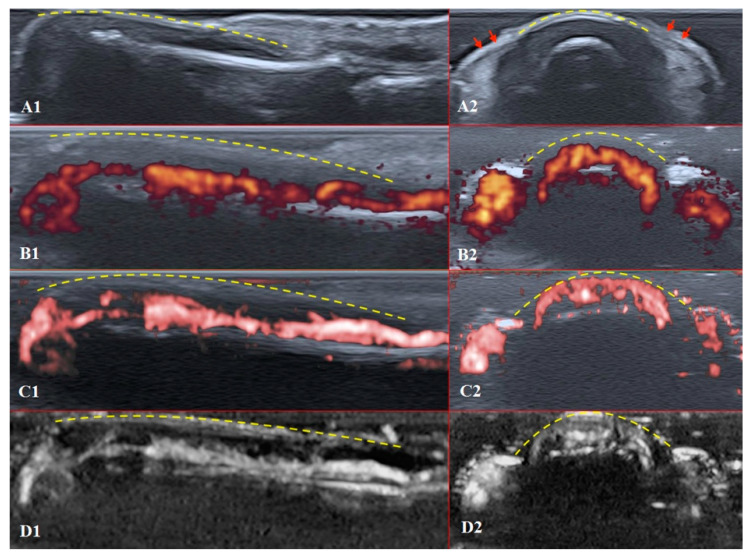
Ultrasound images of the healthy fingertip of the second finger of a hand: (A1,A2) B mode/grayscale; (B1,B2) PD; (C1,C2) cSMI; (D1,D2) mSMI. The yellow dotted lines mark the nail. Sagittal scans (B1–D1) in the midline of the fingertip show vascularity from the deep layers of the nailbed to the end of fingertip pulp. The main landmarks for all transverse scans (B2–D2) are lateral nailfolds (A2 red arrows), which unite in the middle of the proximal nailfold and become a convex line.
